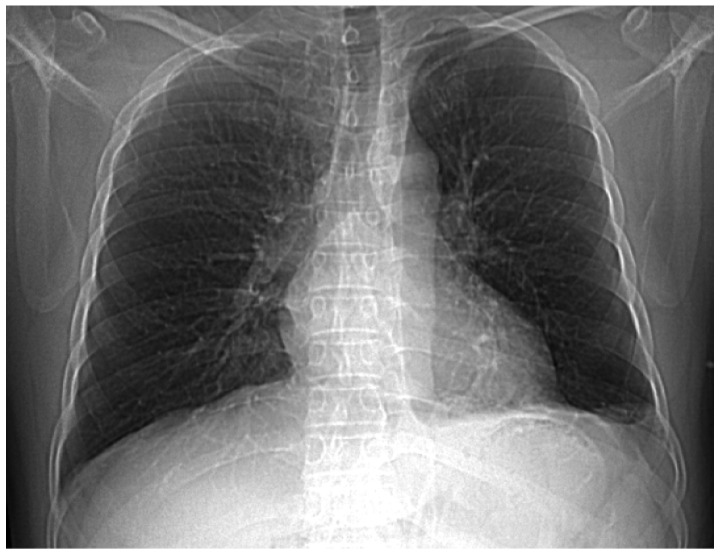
Chest X-ray film on admission showed a dense shadow cord strip in the left lower region.
